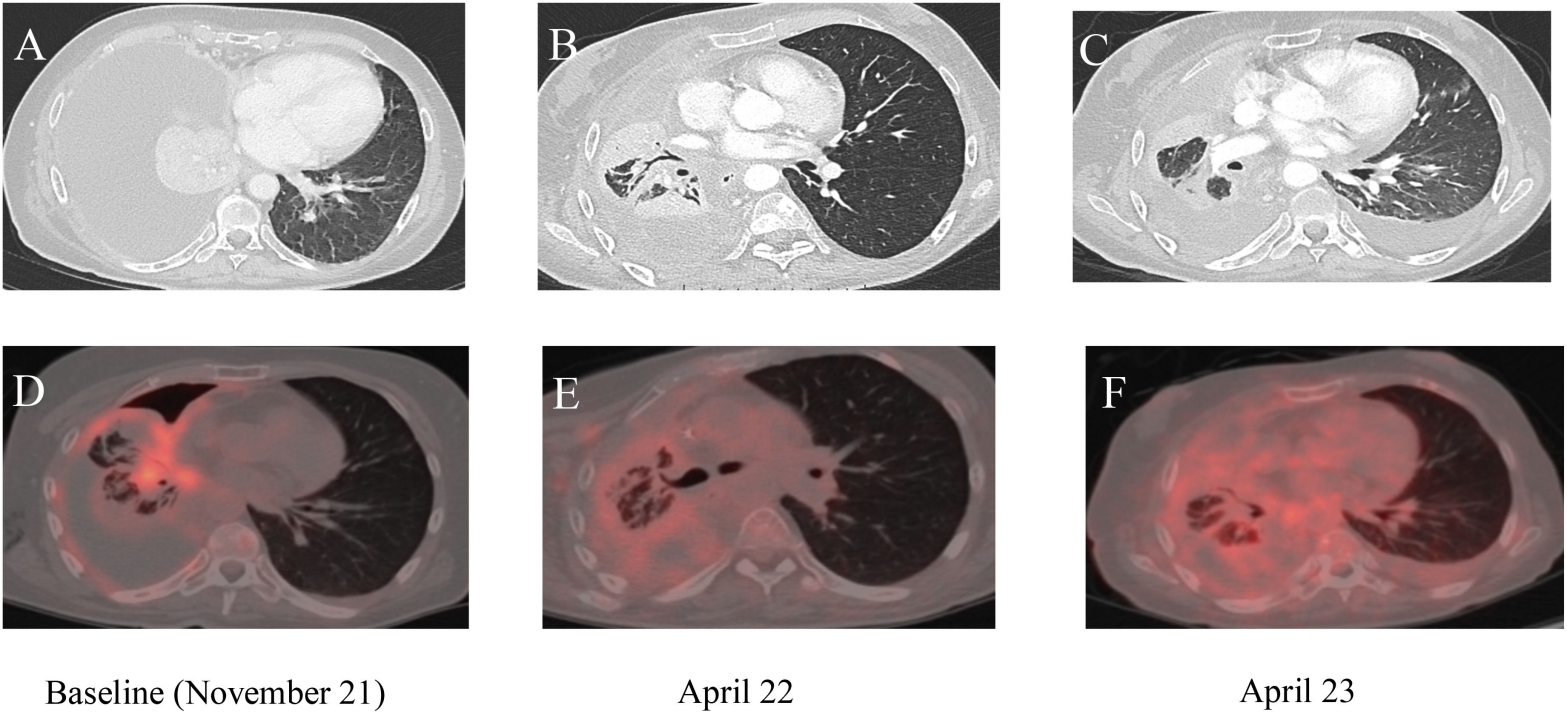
(A–C) Chest computed tomography (CT) images; (D–F) corresponding PET/CT scans. (A, D) Baseline imaging before the start of selpercatinib treatment. (B, E) Imaging results 2 months after the start of treatment. (C, F) Chest CT and PET/CT scans after 14 months of treatment with selpercatinib, highlighting changes in disease progression and response to therapy over time.
